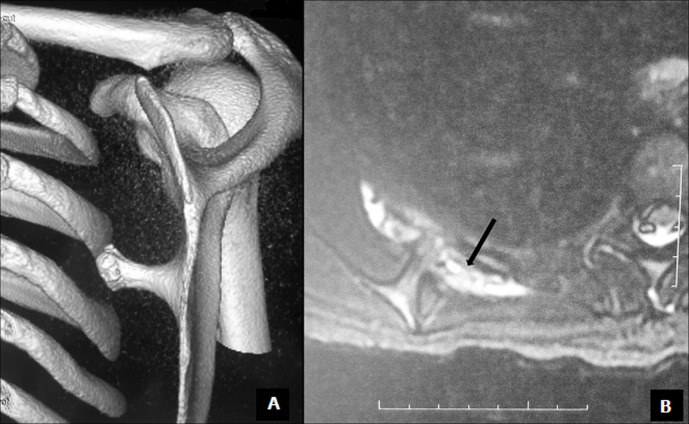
computerized tomography (CT) scanner showing a bony exostosis along the medial border on the ventral surface of the right scapula (A); MRI showing an interscapulo-thoracic bursitis next to the exostosis (B)
